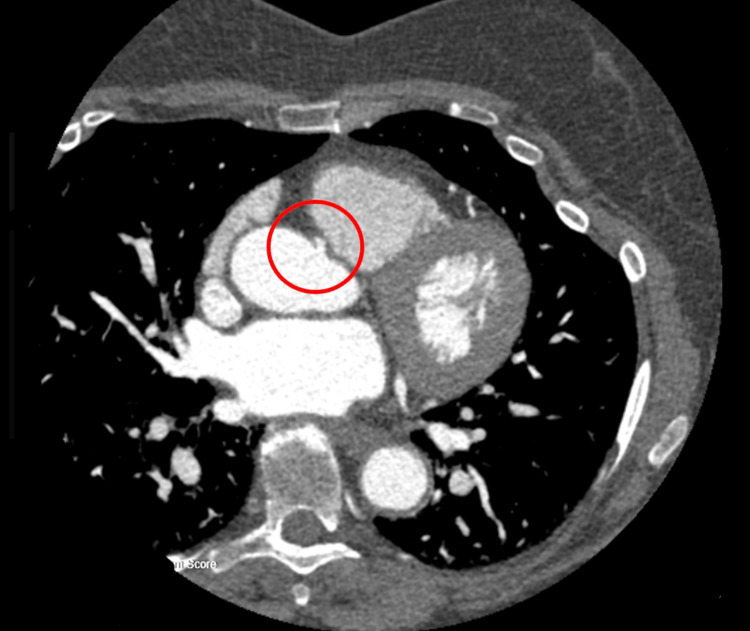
Computed tomography angiography 2D image showing the origin of the anomalous right coronary artery (within the red circle).
